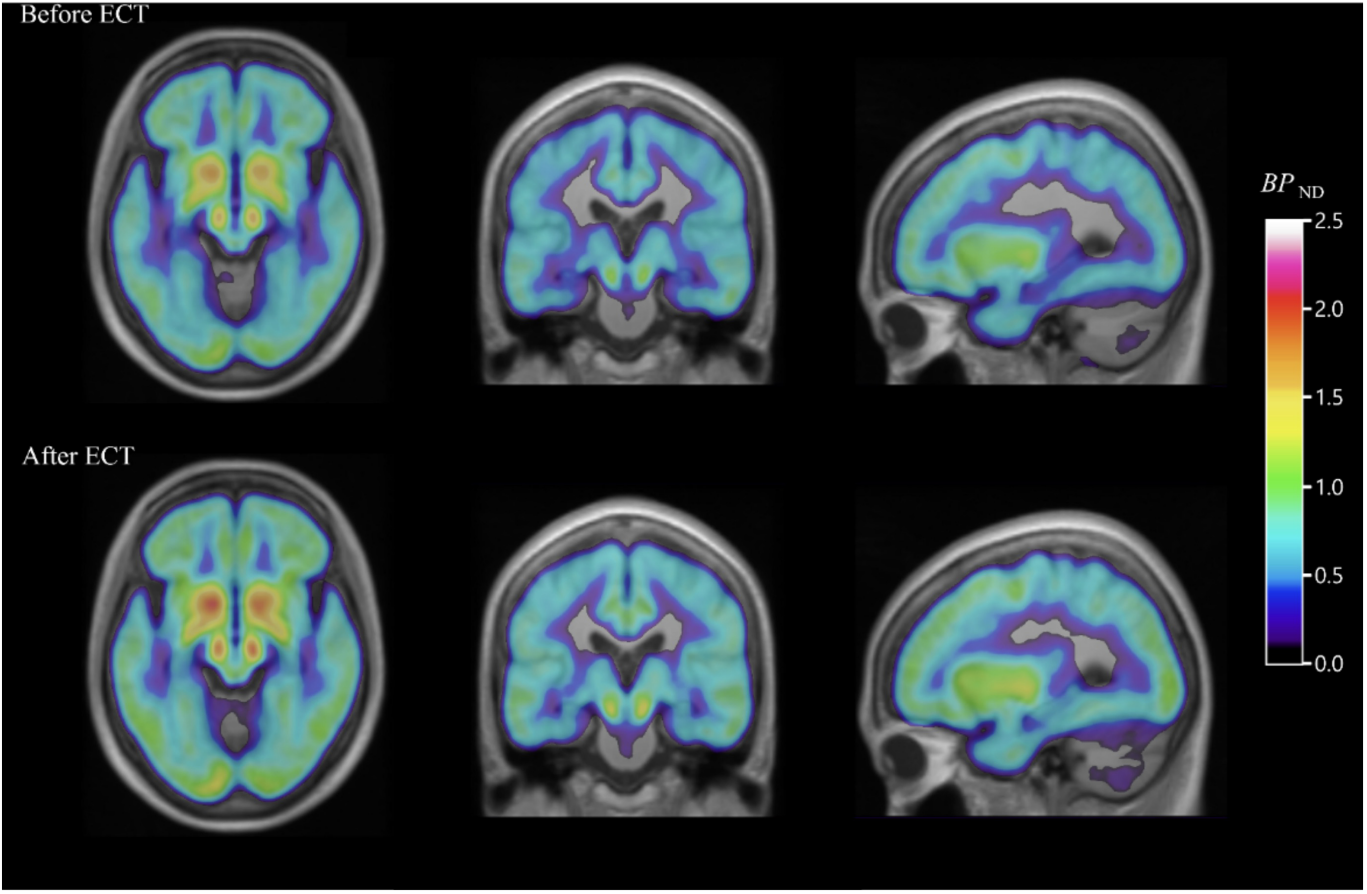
PET images of the serotonin_1B_ receptor effect of electroconvulsive therapy for severe major depressive episodes. Average parametric [^11^C]AZ10419369 PET images overlaid over MR images, showing decreased 5-HT_1B_ receptor binding in the hippocampus before ECT (top) when compared with images taken within one week of (bottom) ECT in major depressive disorder patients [with permission from reference (81)].
